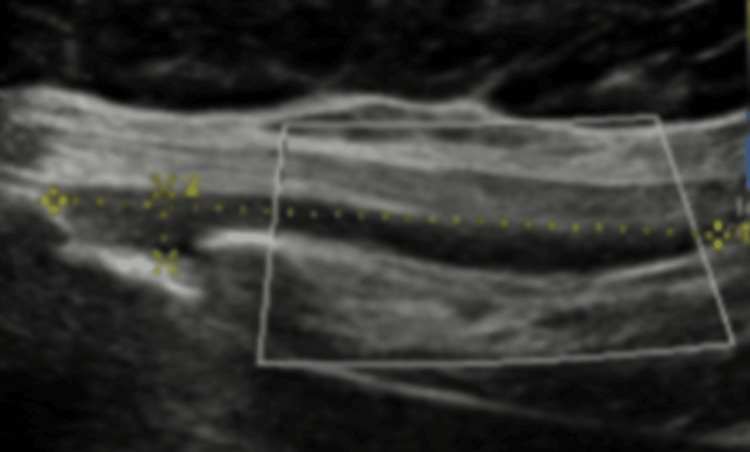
Further reduction of chest wall lesion at two-month follow-up. Follow-up ultrasound of the right anterior chest wall at two months demonstrates a small residual hypoechoic collection measuring approximately 4.1 × 0.5 × 0.5 cm (estimated volume ~0.5 mL). The collection shows a further reduction in size compared with the prior study.
